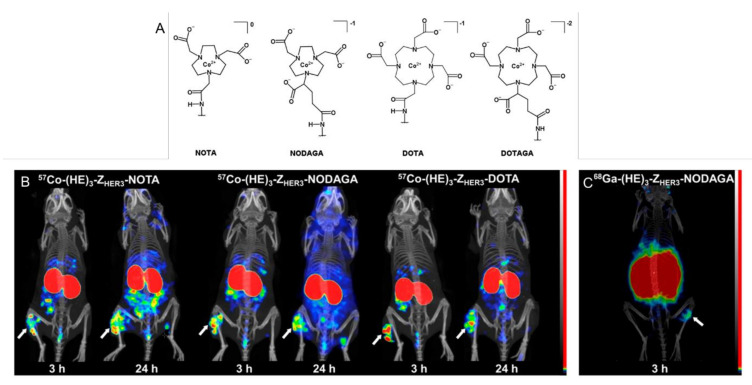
Chemical structures and charges of studied cobalt-chelate complexes (A). SPECT/CT images of the [^57^Co]Co-HEHEHE-Z_HER3_-X affibody with NOTA, NODAGA, or DOTA chelator (B). For each agent tested, the later imaging timepoint produced better tumor contrast. PET/CT of [^68^Ga]Ga-HEHEHE-Z_HER3_-NODATA was only possible to 3 h PI and showed greater kidney accumulation (C) [135].
